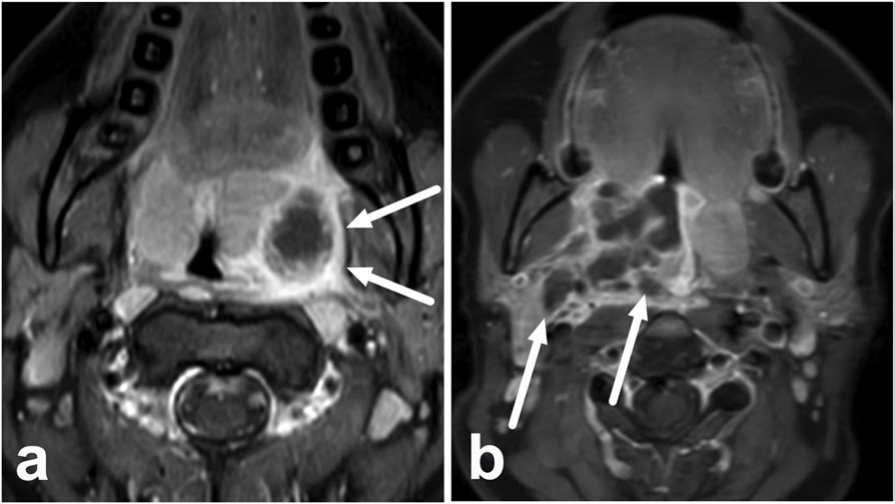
Examples of a simple peritonsillar abscess (a) and a deeply extending abscess (b) on axial fat-suppressed postcontrast T1-weighted Dixon images in different patients. Abscesses present as areas of non-enhancement in these images. In the case of a peritonsillar abscess, the abscess is contained within the enhancing superior constrictor muscles (arrows, a). In the deeply extending abscess, non-enhancing abscess material (arrows) can be seen extending into the parapharyngeal space, far lateral from the muscle border (b)
